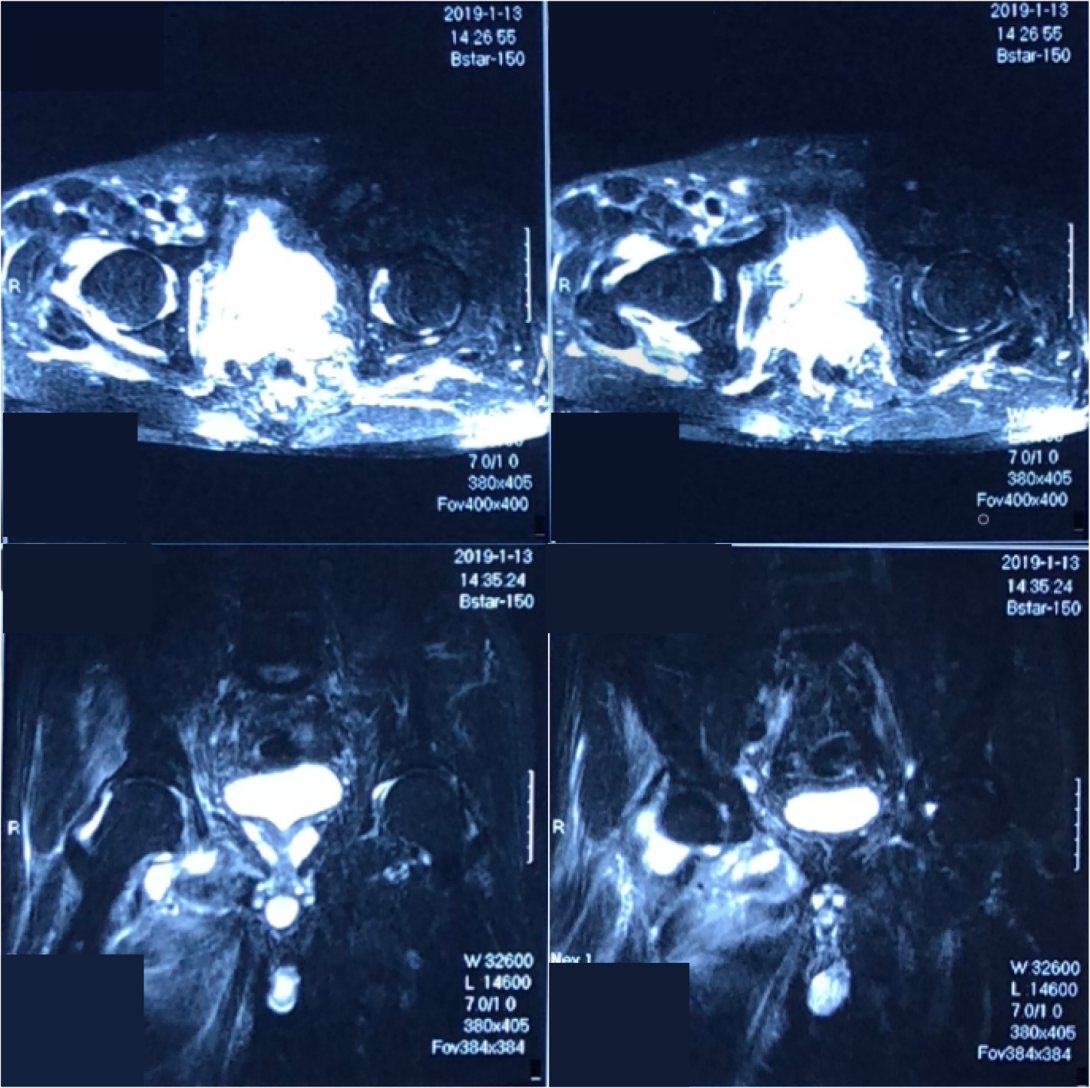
MRI of the right hip demonstrated an effusion and synovitis in the joint
